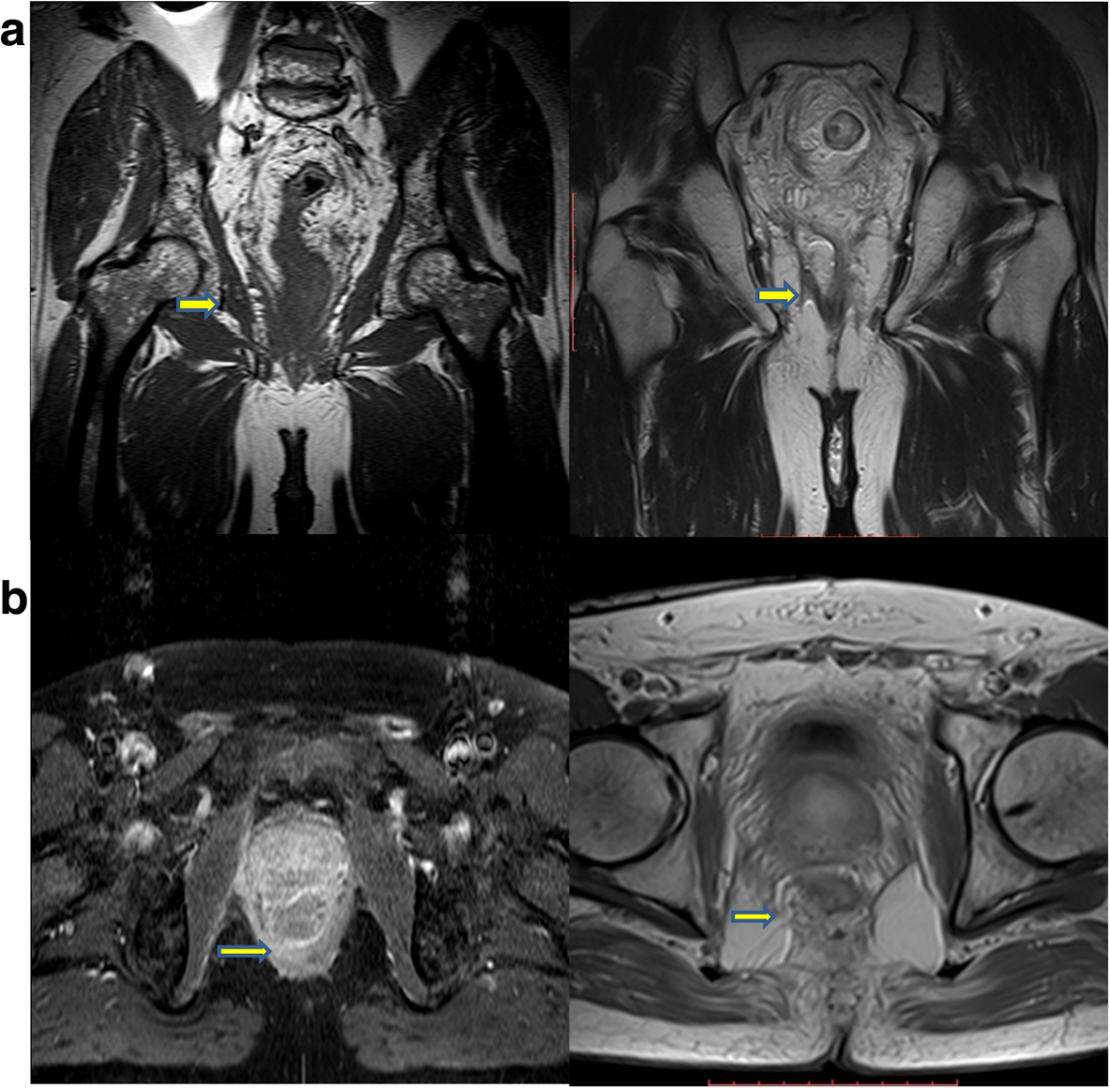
a MRI coronal view of patient pelvis pre- and post operatively (left and right, respectively) (arrow shows the infiltration of right portion of levetor ani muscle by the tumor and the defect remaining after the partial excision of levator muscle ani). Preoperative MRI was performed after neoadjuvant treatment (seventh week). b MRI axial view of the same patient’s area of interest
